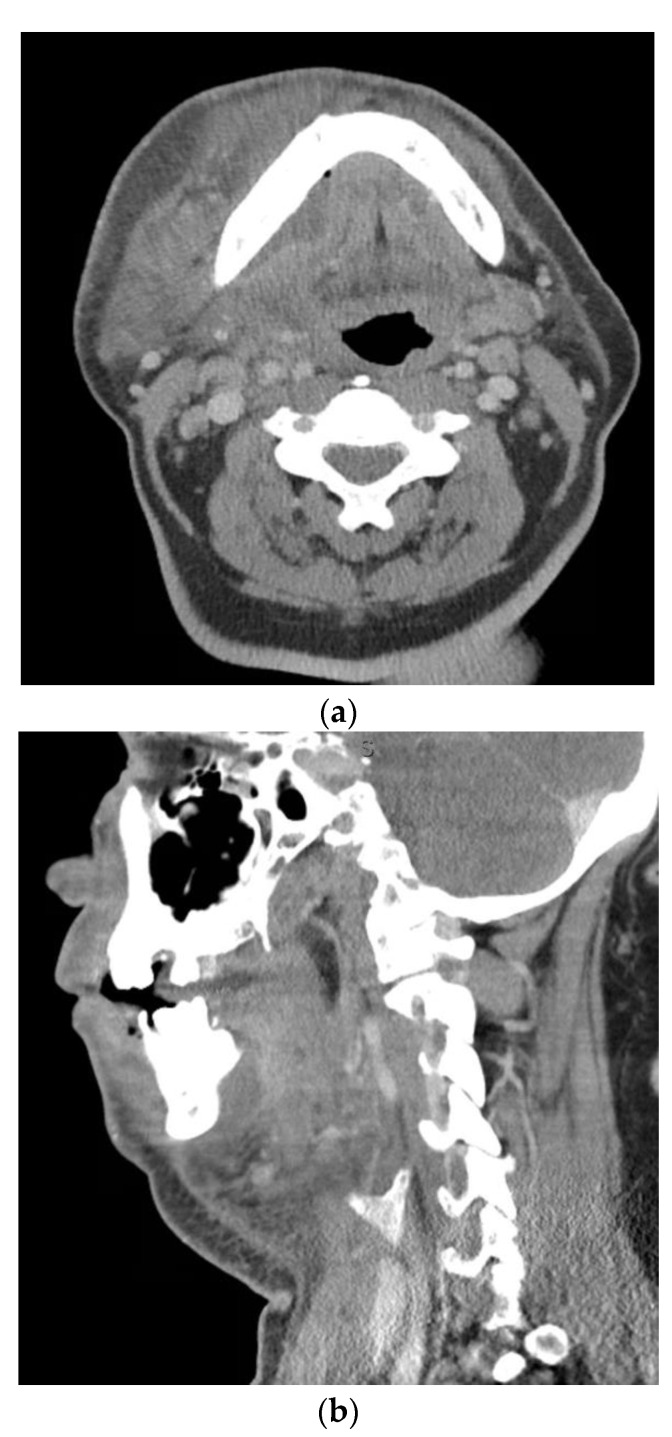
Axial (a) and sagittal (b) computed tomography scan views of patient with right submandibular, sublingual, and submental abscess with associated odontogenic severity score of six.
